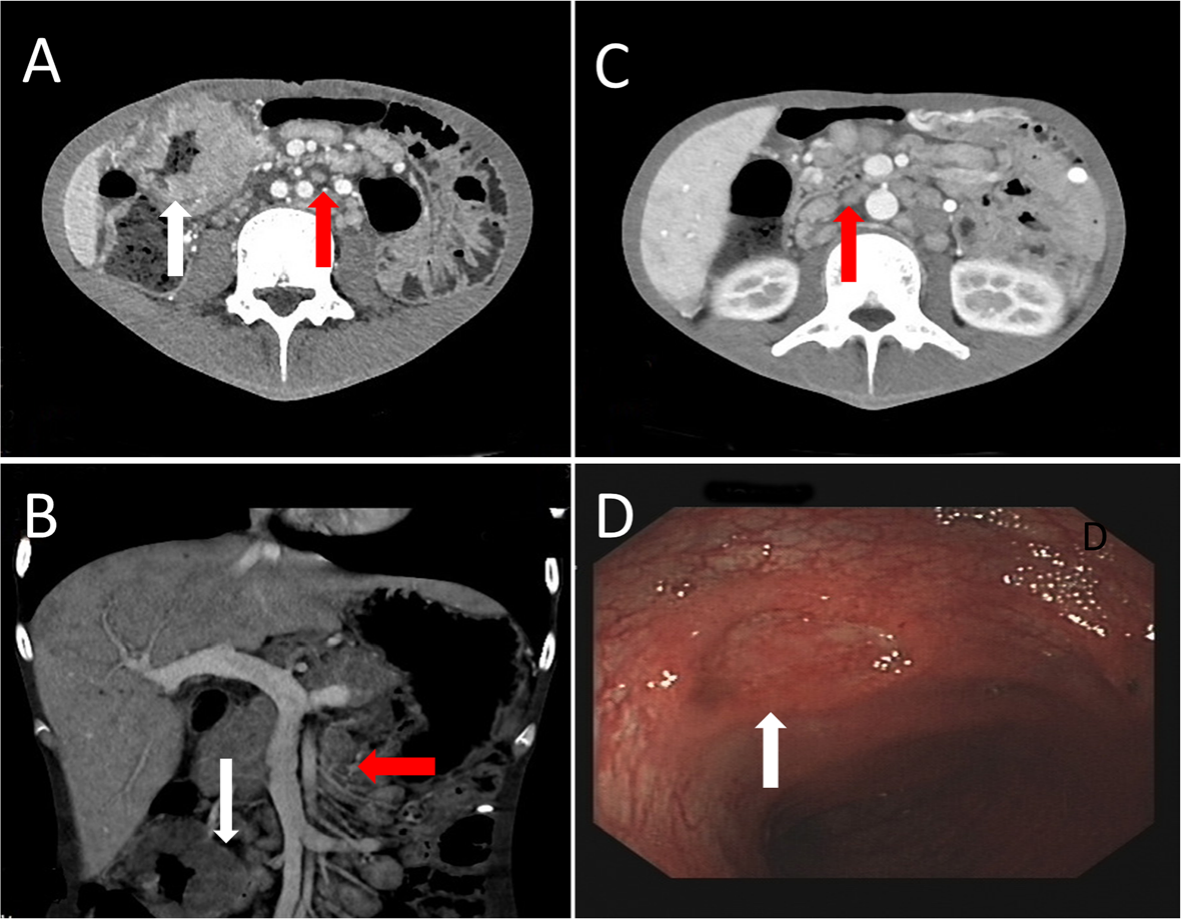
Enhanced abdominal CT (a, b, c) showed significant lymph node enlargement (red arrows) and multiple thickening of the intestinal wall (white arrows). Colonoscopy indicated multiple ulcerations of the colon (d, white arrow)
